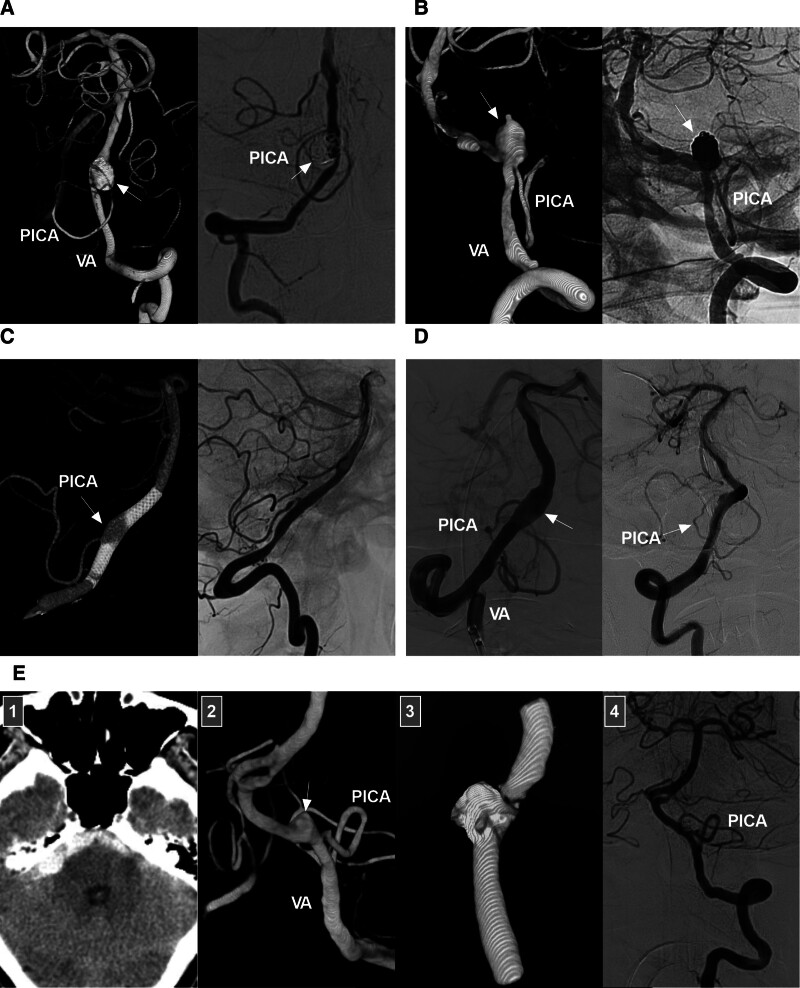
Reconstructive treatment for VA dissecting aneurysms with PICA involvement. (A) Traditional stent-assisted coiling: follow-up 3-dimensional (left panel) and 2-dimensional (right panel) DSA images showing complete occlusion of a sidewall VA trunk dissecting aneurysm (arrow) with PICA involvement; the PICA has been preserved. (B) Traditional stent-assisted coiling: left panel: 3-dimensional DSA image showing a saccular VA trunk aneurysm (arrow) with PICA involvement; right panel: unsubtracted DSA image showing that the aneurysm (arrow) has been coiled with stenting assistance; the PICA has been preserved. (C) FD deployment alone: left panel: reconstructive DSA image showing coverage of the aneurysm (arrow) by an FD; the PICA is patent; right panel: 1-year follow-up DSA image showing cure of the dissecting aneurysm and preservation of the PICA. (D) FD deployment alone: left panel: DSA image showing a VA dissecting aneurysm (arrow) following FD coverage; the PICA (arrow) is patent; right panel: 6-month follow-up DSA image showing reconstruction of the VA, regression of the aneurysm (arrow), and patency and thinning of the PICA. (E) FD deployment with adjunctive coiling: panel 1: Computed tomography image showing subarachnoid hemorrhage (asterisk); panel 2: 3-dimensional DSA image showing a VA dissecting aneurysm (arrow), with the PICA (arrowhead) emerging from the proximal portion of the aneurysm; Panel 3: Image showing the FD device and coils; Panel 4: 6-month follow-up DSA image showing cure of the aneurysm and patency of the PICA. DSA = digital subtraction angiography, FD = deployment, PICA = posterior inferior cerebellar artery, VA = vertebral artery.
